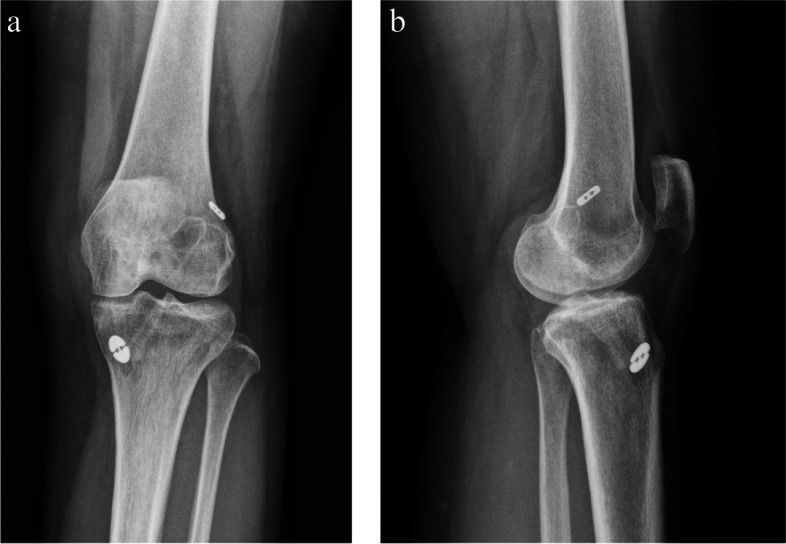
a Anteroposterior view of radiograph of ASIS hybrid tibial fixation at least 1 year after operation. b Lateral view of radiograph of ASIS hybrid tibial fixation at least 1 year after operation
